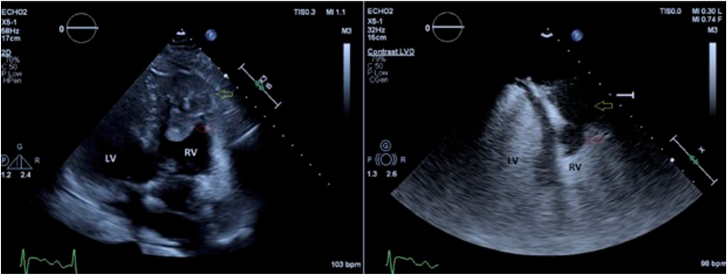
Preprocedural transthoracic echocardiographic imaging. (Left) Apical 4-chamber view demonstrating a large, well-demarcated, echodense mass involving the right ventricle (RV) lateral wall and apex (yellow arrow), with an adjacent, more homogeneous echogenic thrombus (red arrow) in the RV apex. (Right) Contrast-enhanced apical 4-chamber view improves delineation of both the RV mass (yellow arrow) and the associated thrombus (red arrow), confirming their intracavitary extension.
